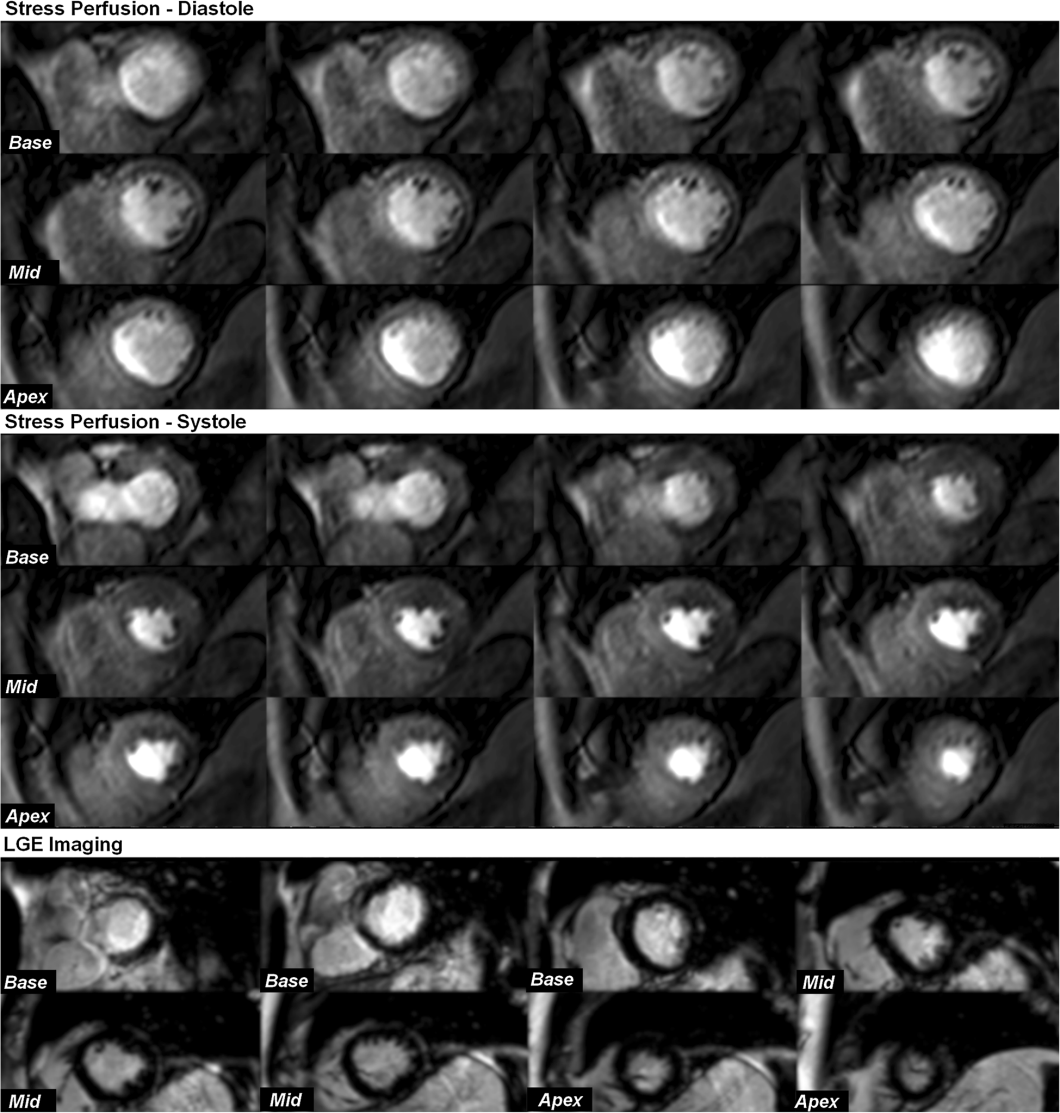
Case example: 3D-perfusion CMR in systole and diastole. This example shows 3D-perfusion CMR in a 75-year-old man with angina. Stress-induced perfusion defects are seen infero-laterally from base to apex and antero-laterally from mid-ventricle to apex in both diastole and systole. However, perfusion defects are difficult to discern from dark-rim artifact in diastole and are more clearly delineated with systolic acquisition. Late-gadolinium enhancement imaging did not reveal any myocardial infarction. X-ray coronary angiography revealed 80% stenosis of a large diagonal branch and significant proximal disease in a large dominant left circumflex artery.
